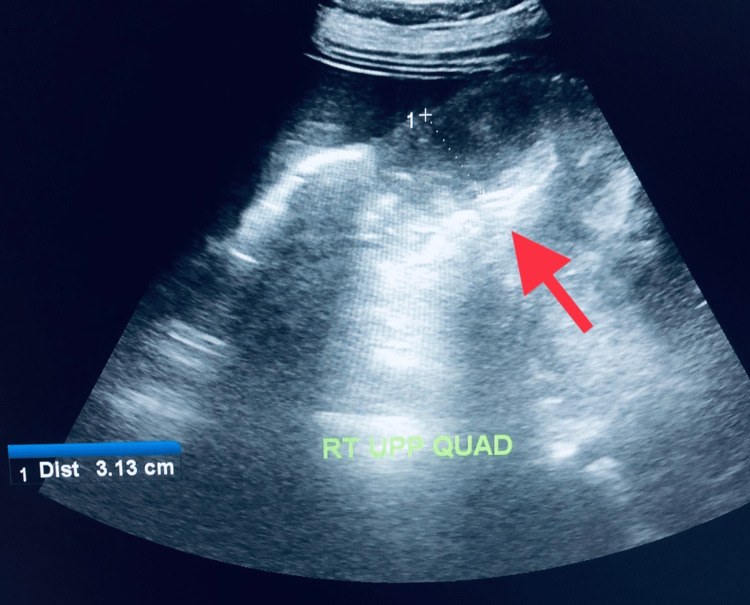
Ultrasound of the abdomen The image shows dilated small bowel loops with a diameter of more than 3 cm (red arrow).
